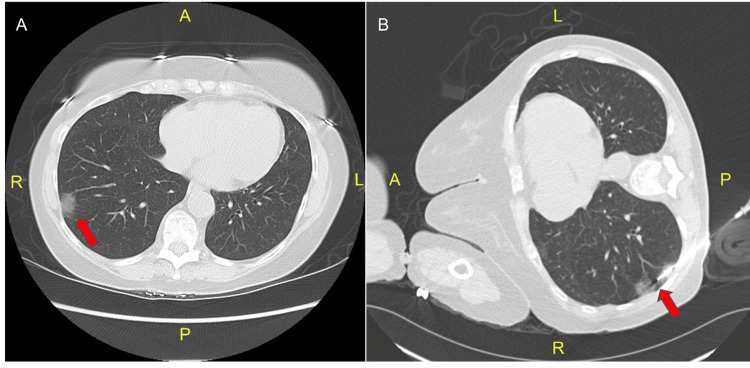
A) Chest computed tomography axial view showing a 23 x 14 mm ground-glass nodule in the right lower lobe. B) Computed tomography image obtained during a CT-guided biopsy, showing a biopsy needle targeting the ground-glass nodule in the right lower lobe.
